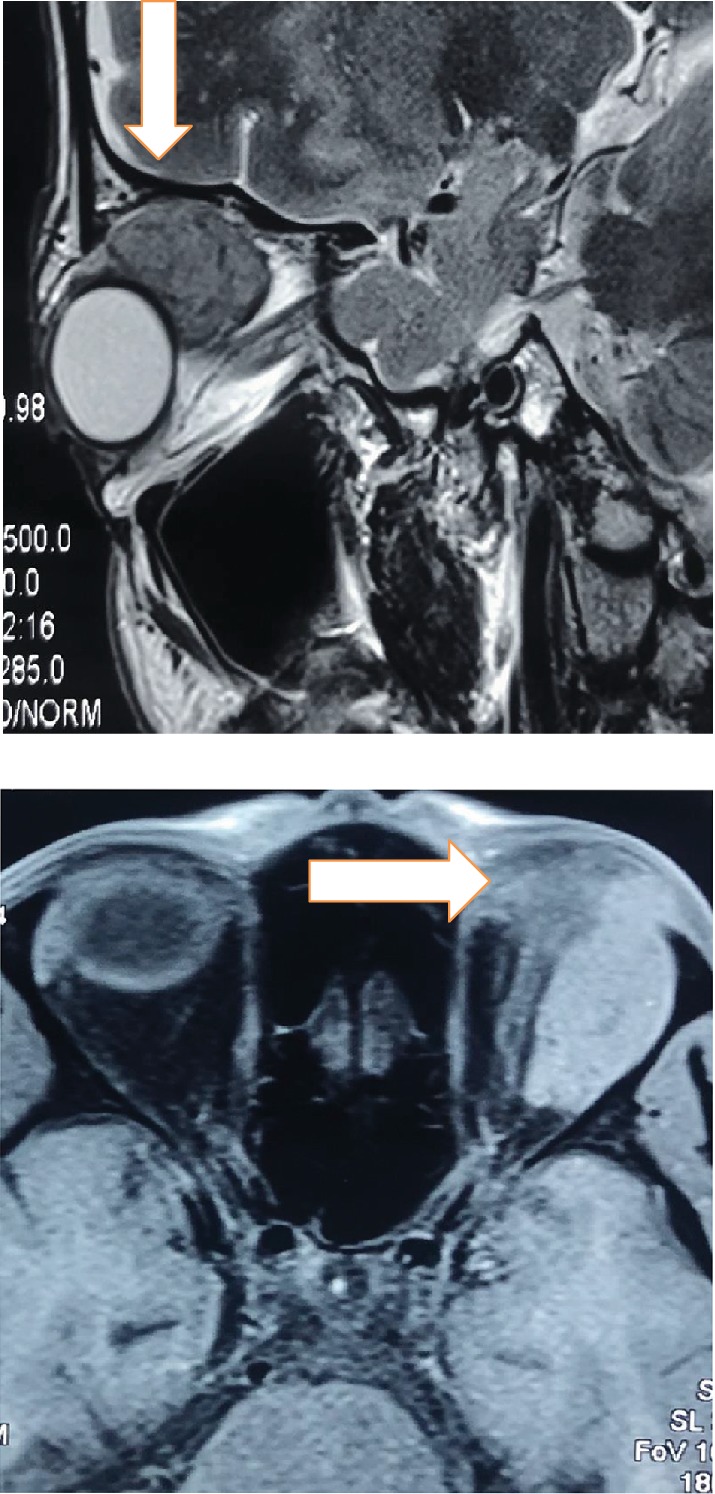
CE-MRI (Contrast-Enhanced Magnetic Resonance Imaging) showing left well-defined orbital lesion in the extraconal compartment abutting the left lateral rectus muscle with intraconal extension.
